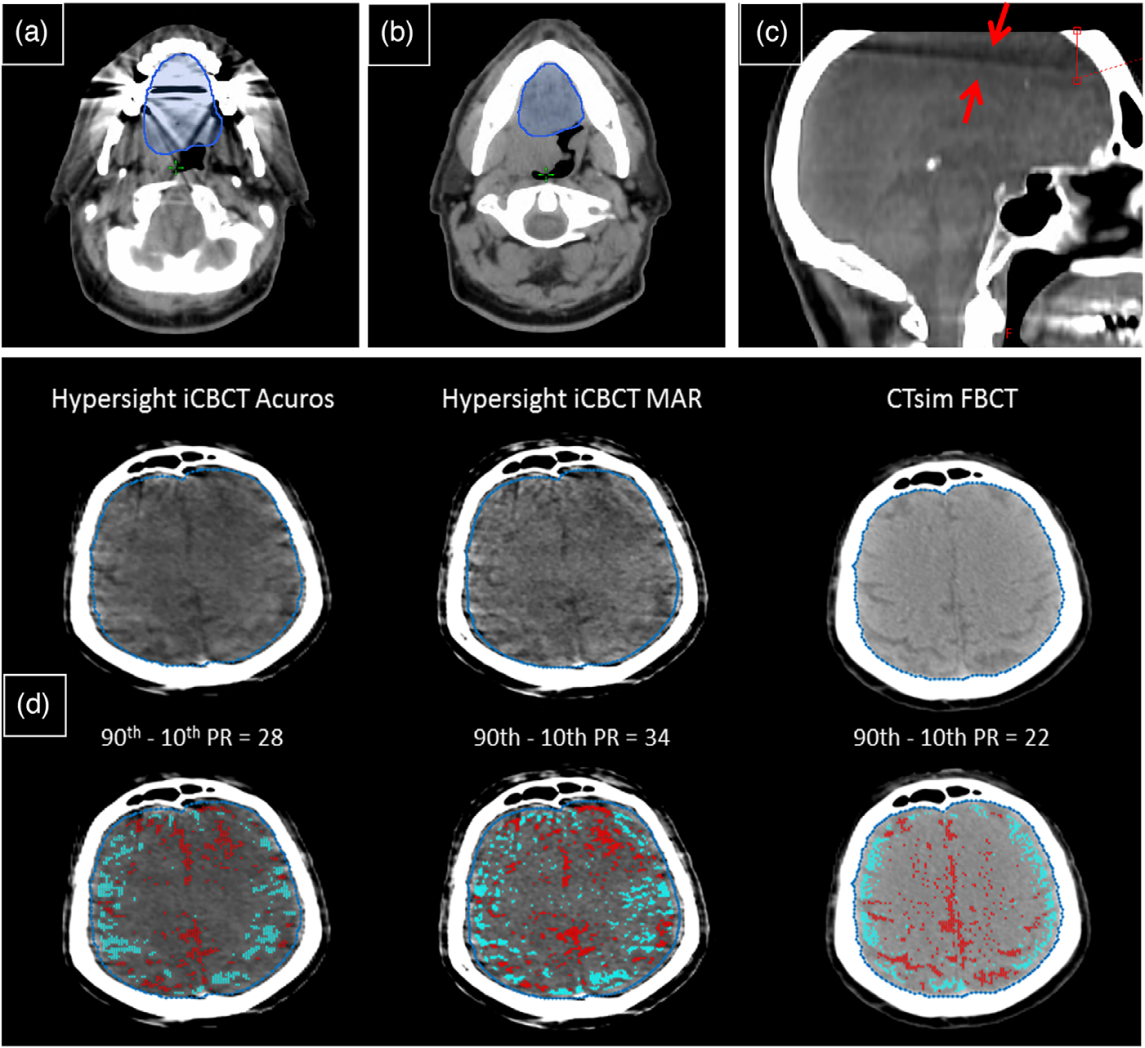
The slices used to define (a) the artifact ROI and (b) the background ROI, for calculating the artifact index. Both slices are from the same FBCT image of a single patient, with the oral cavity structure shown in blue. (c) Shows the cone‐beam effect artifact in the HyperSight iCBCT Acuros image, and (d) is the slice selected to evaluate the brain percentile range (PR), displayed with the same window width and level settings. The PR is recorded for this patient as an example, and the bottom row of (d) shows pixels with HU above the 90^th^ percentile in that slice colored in red, and pixels with HU below the 10^th^ percentile in teal.
